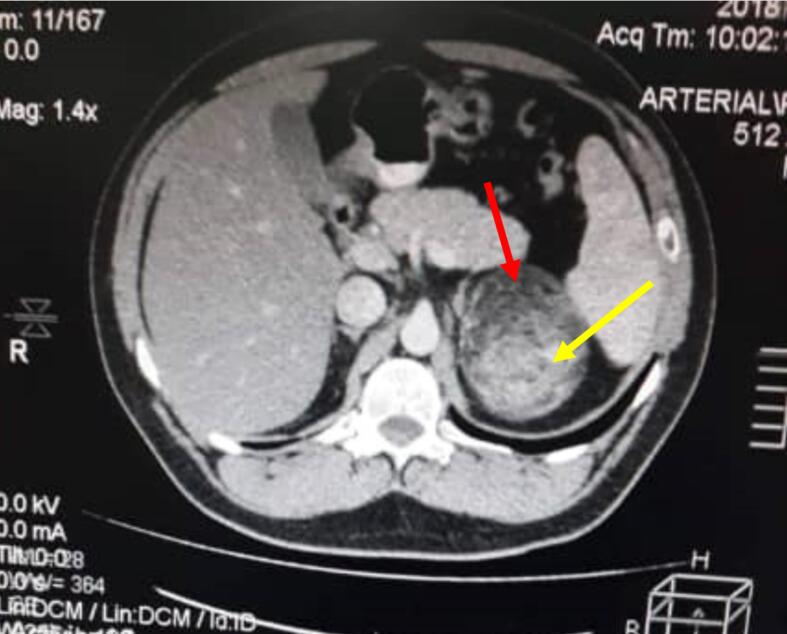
Contrast enhanced axial abdominal CT scan showing the unilateral AML mass. The mass is heterogenous due to mixed composition. Red arrow shows the fat component which appears hypodense (darker) on the CT scan, while the yellow arrow shows the hyperdense vascular and muscle tissue (brighter) on CT scan due to uptake of contrast material. The mass is well-circumscribed, the perimeter can be traced without discontinuations. (For interpretation of the references to colour in this figure legend, the reader is referred to the web version of this article.)
